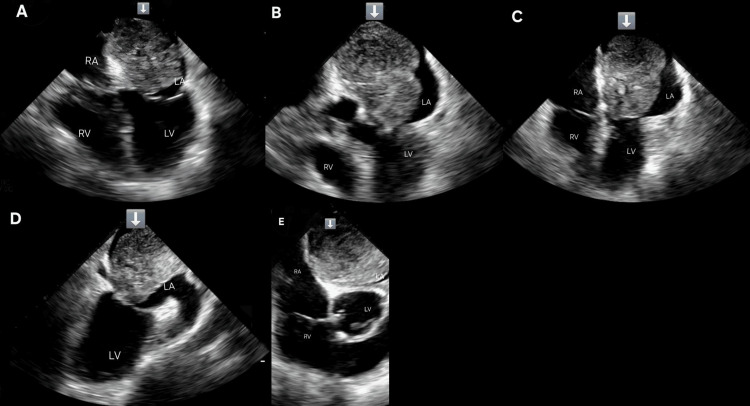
Multimodal images of transesophageal echocardiographic assessment of left atrial mass (A-C) Mid-esophageal four-chamber view showing the myxoma in the left atrium. (D) Mid-esophageal long-axis view showing the myxoma in the left atrium causing outflow obstruction. (E) Mid-esophageal long-axis view showing the large atrial myxoma associated with severe mitral flow obstruction, with a mean gradient of 15 mmHg
